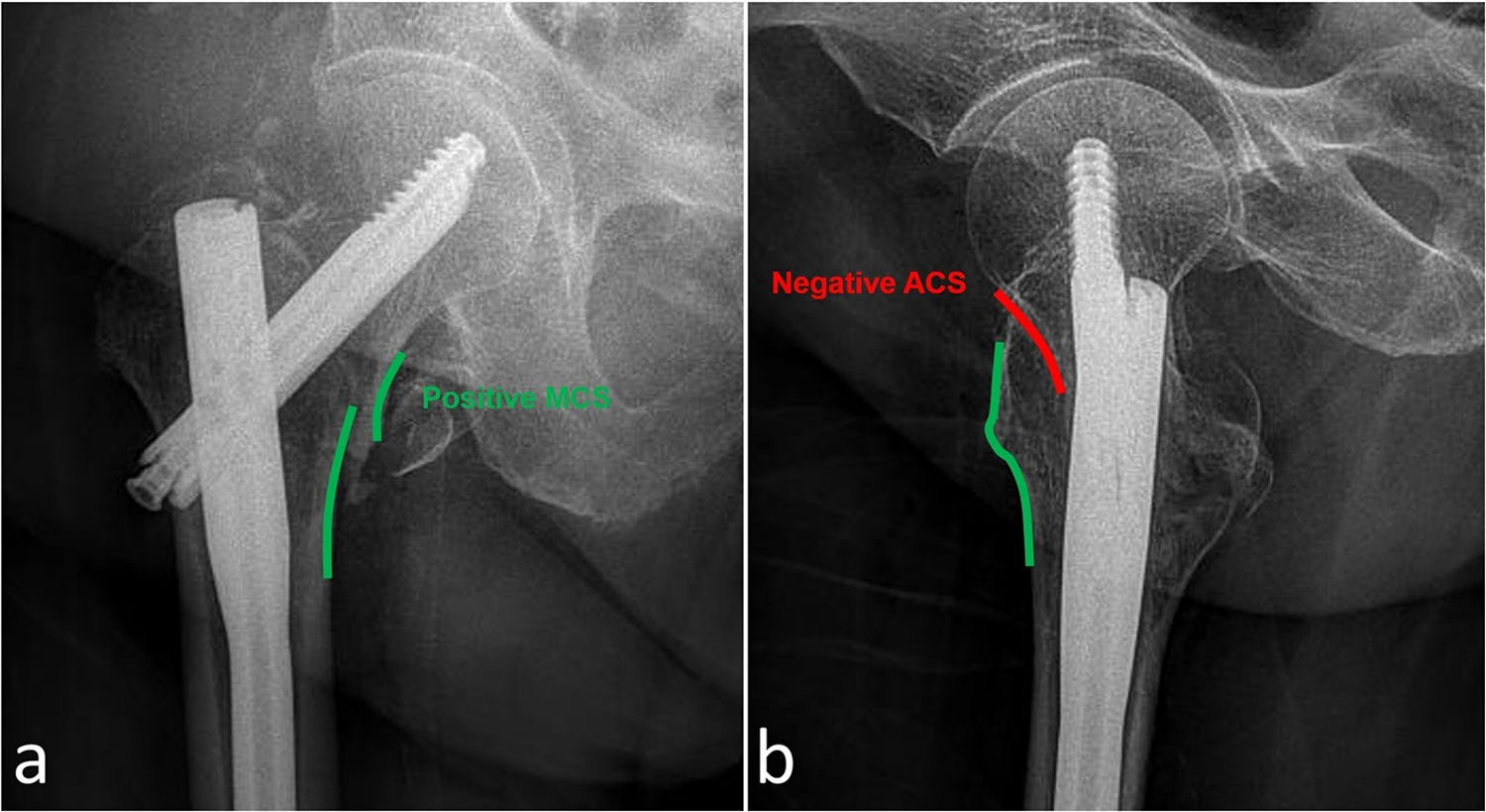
Examination of cortical support between fracture fragments to assess the reduction quality. Positive medial cortical support on AP radiograph (a). Negative anterior cortical support on the lateral radiograph (b)
